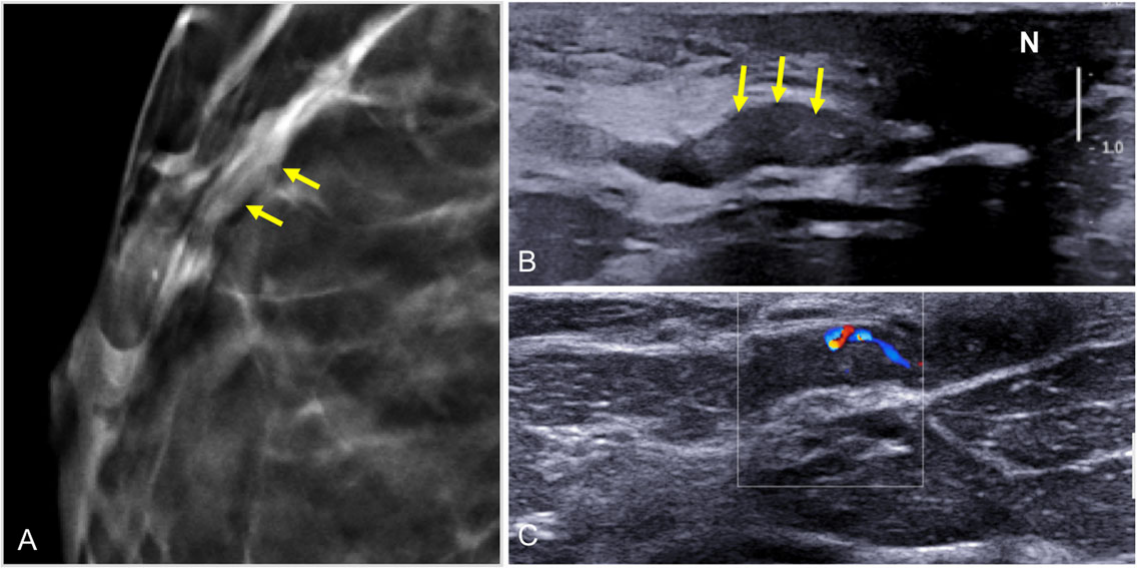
Ductal ectasia with intraductal contents. Solitary intraductal papilloma. A 47-year-old woman with serous secretion from a single orifice in the right nipple. a Craniocaudal tomosynthesis slice shows a nodular image with well-defined borders in the retroareolar region of the outer quadrants with a segmental distribution (arrows). b Ultrasound shows ductal ectasia with a well-defined solid nodular lesion (arrows) adjacent to the nipple (N). c Doppler signal due to flow inside the intraductal lesion
